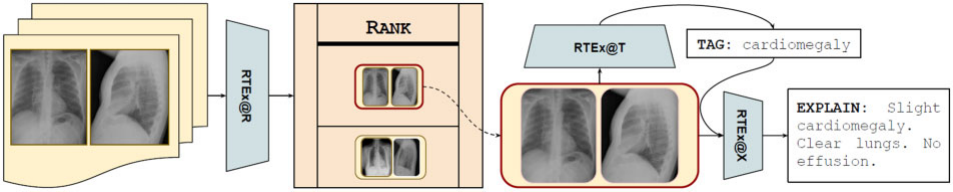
A depiction of our RTEx framework. It first ranks the radiography exams based on their probability (ie, using the radiographs of each exam) to include an abnormality. The highest ranked are tagged with abnormality terms and an explanatory diagnostic text is automatically provided to assist the expert.
